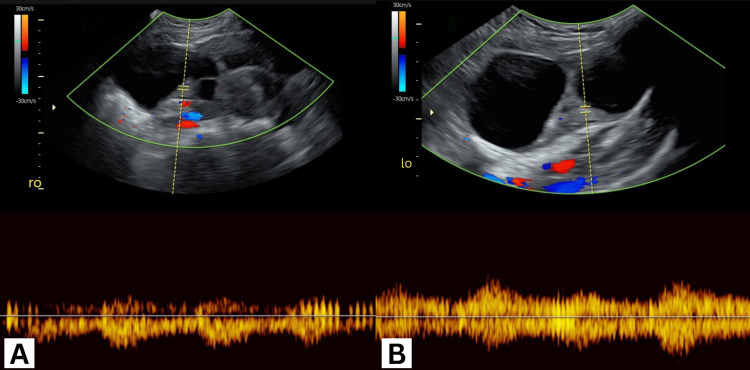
Doppler waveform demonstrating adequate blood flow to the; (A) right ovary (ro) and (B) left ovary (lo).
